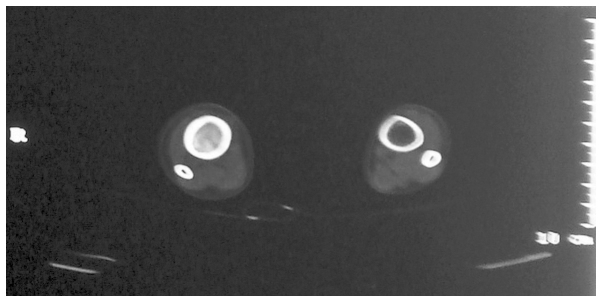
Computed tomography of the tibia showed a narrow medullary cavity packed with high density osteoid tissues.
